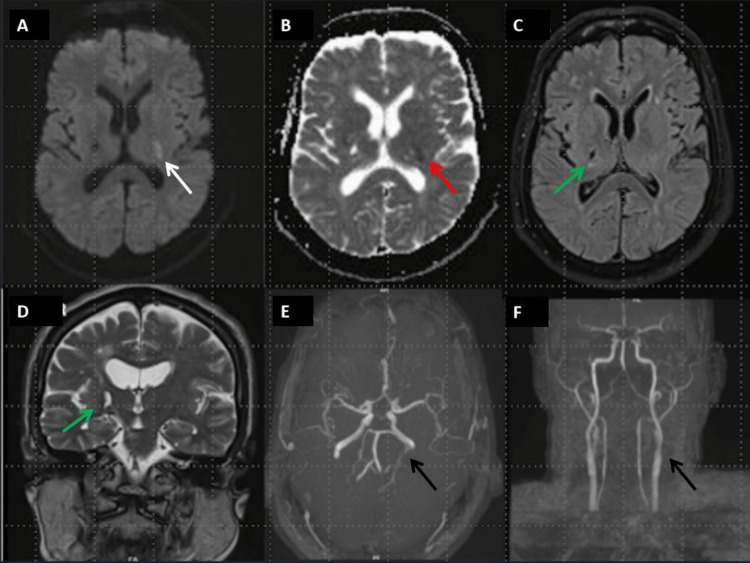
MRI brain showing (A, B) diffusion restriction in left capsuloganglionic region on DWI (white arrow), with corresponding ADC (red arrow) suggesting acute infarct; (C, D) FLAIR axial sequence showing hypointensity in right capsuloganglionic region and hyperintensity in T2 coronal sequence suggesting gliosis secondary to old stroke (green arrows); (E, F) MRA showing normal cranial vessels (black arrows). MRI: magnetic resonance imaging; DWI: diffusion-weighted imaging; ADC: apparent diffusion coefficient; FLAIR: fluid-attenuated inversion recovery; MRA: magnetic resonance angiography
